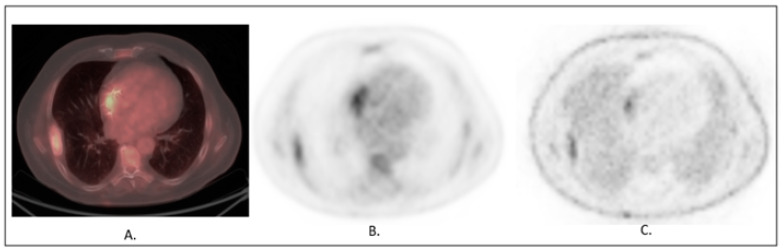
Infective Endocarditis in a patient with a cardiac device-related infective endocarditis. Axial scans at the level of the pacing leads: Infection focus in the right atrial leads. (A) Fused ^18^FDG PET and CT with tracer uptake in right atrium. (B) ^18^FDG PET attenuation-corrected scan. (C) ^18^FDG PET attenuation non-corrected scan showing uptake presence matching attenuation-corrected scan.
